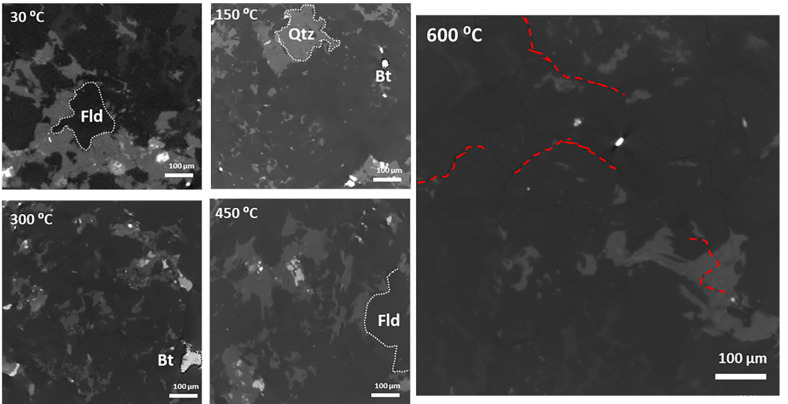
XY slices of X-ray CT images of thermally treated granite samples. The dominant compositions i.e. quartz (Qtz), feldspars (Fld) and biotite (Bt) has been shown in the images. Thermal cracks are distinctly visible at 600 °C and are marked with red dotted lines.
